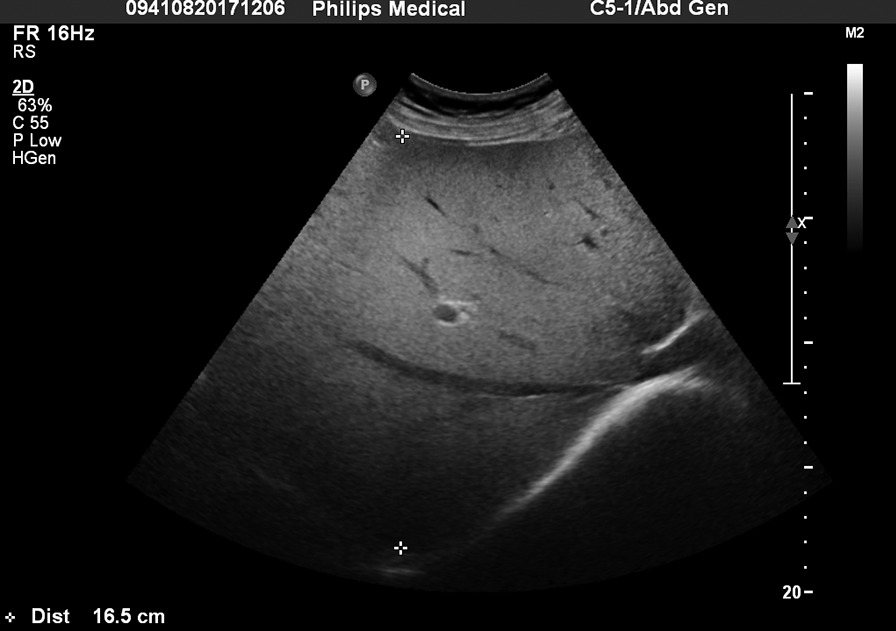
Abdominal ultrasound showed an enlarged, fatty liver with enhanced liver parenchymal echogenicity
